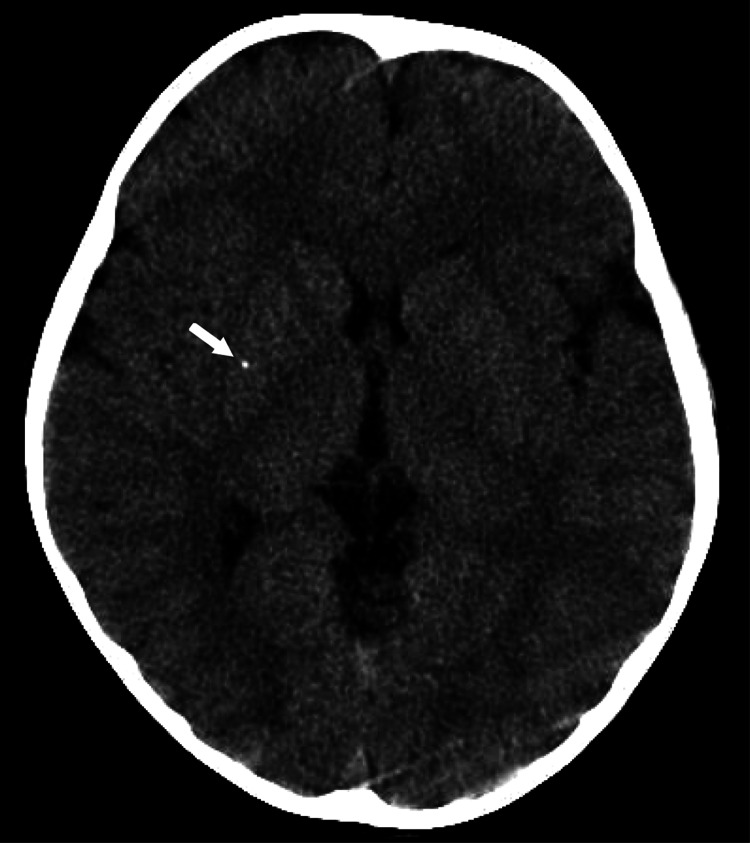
Head computed tomography (CT) scan on day one. There was a motion effect associated with body movement, though there was no obvious bleeding or fractures. The head CT taken on the first day was later reviewed to find mineralizing angiopathy (arrow) at the right basal ganglia.
